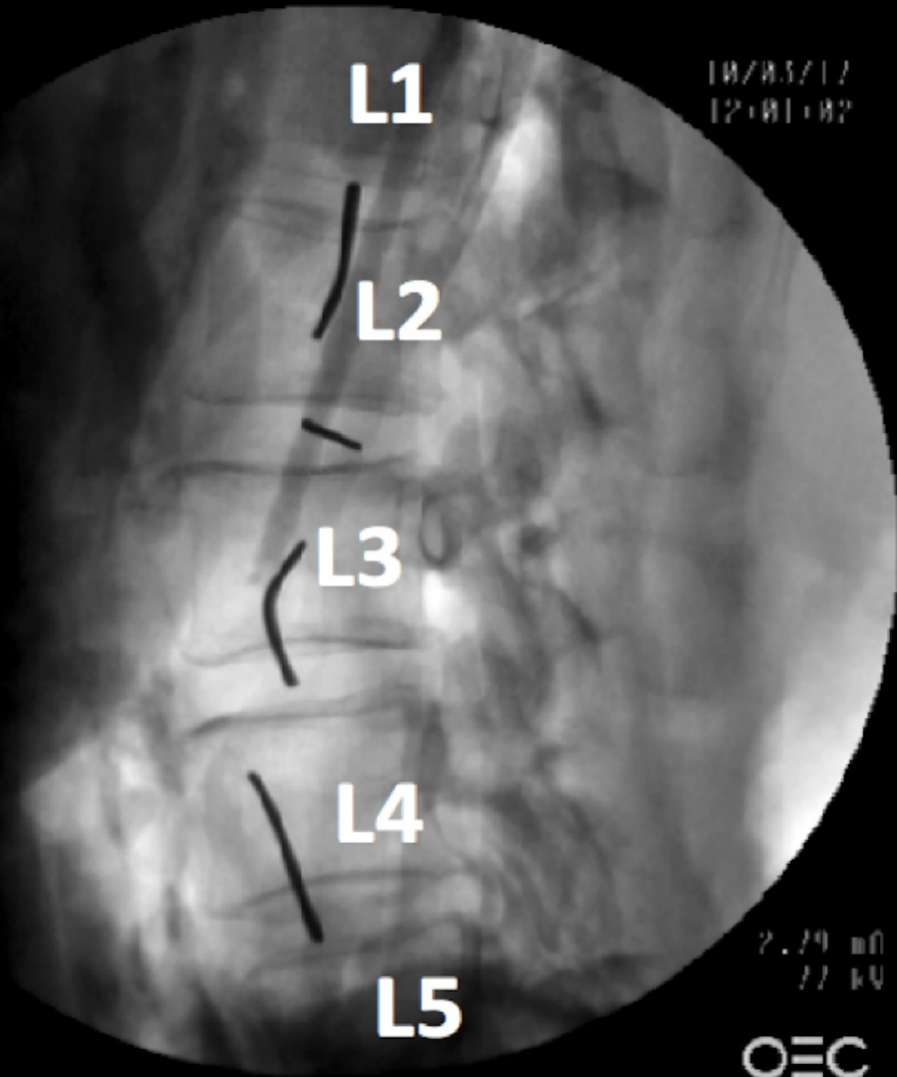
Labeled lateral radiograph of the lumbar spine showing the placement of the wires which were used for the measurements.
